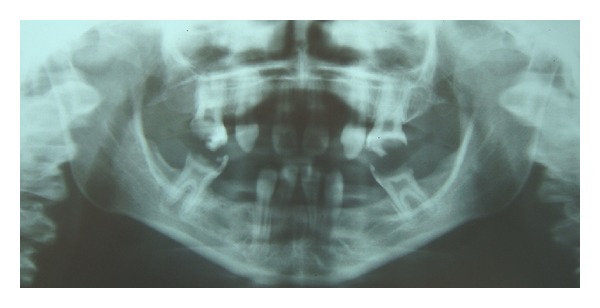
Oligodontia is clearly observed on panoramic radiograph.
